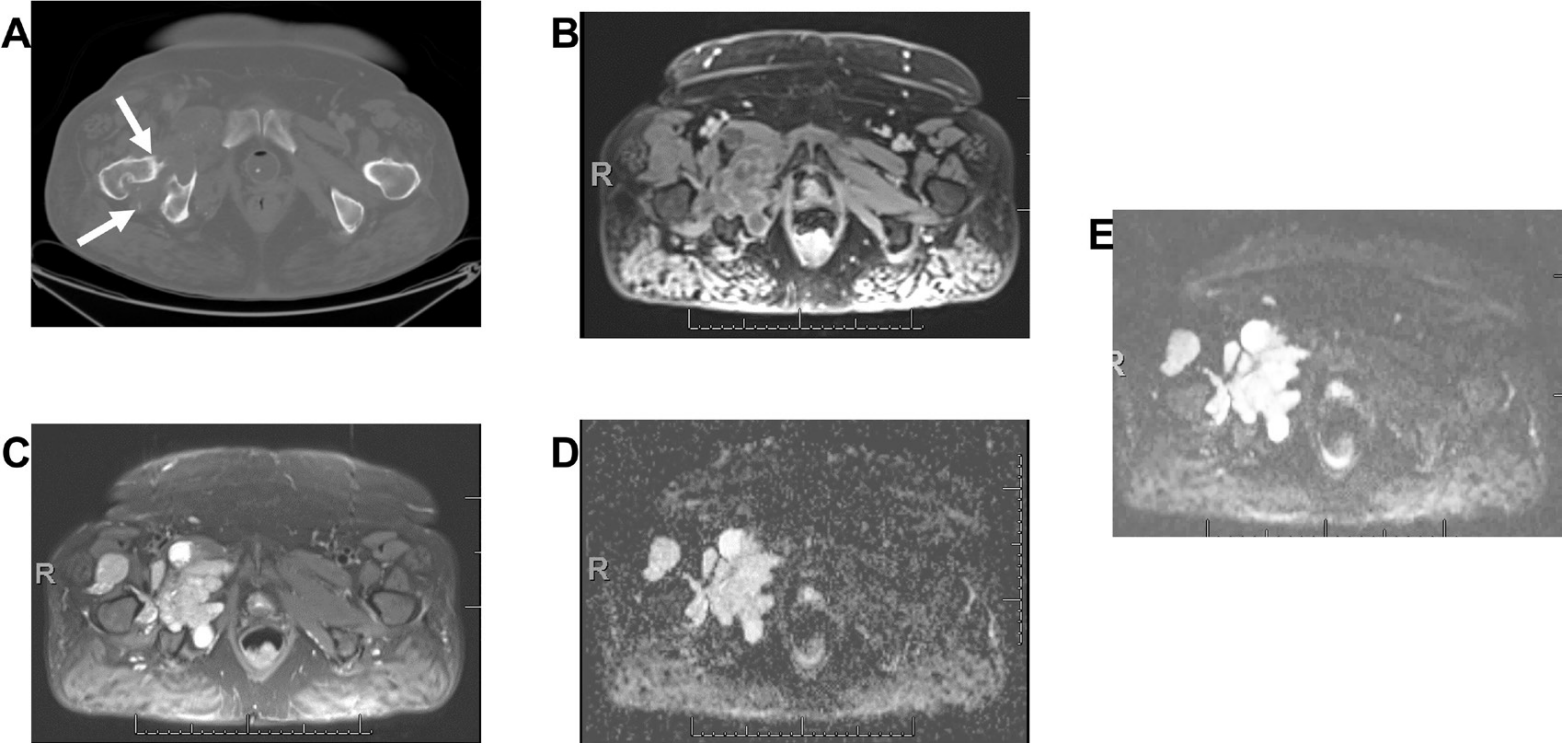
Synovial chondromatosis with low-grade chondrosarcoma on axial CT of pelvis (arrows) (A), fs*T*
